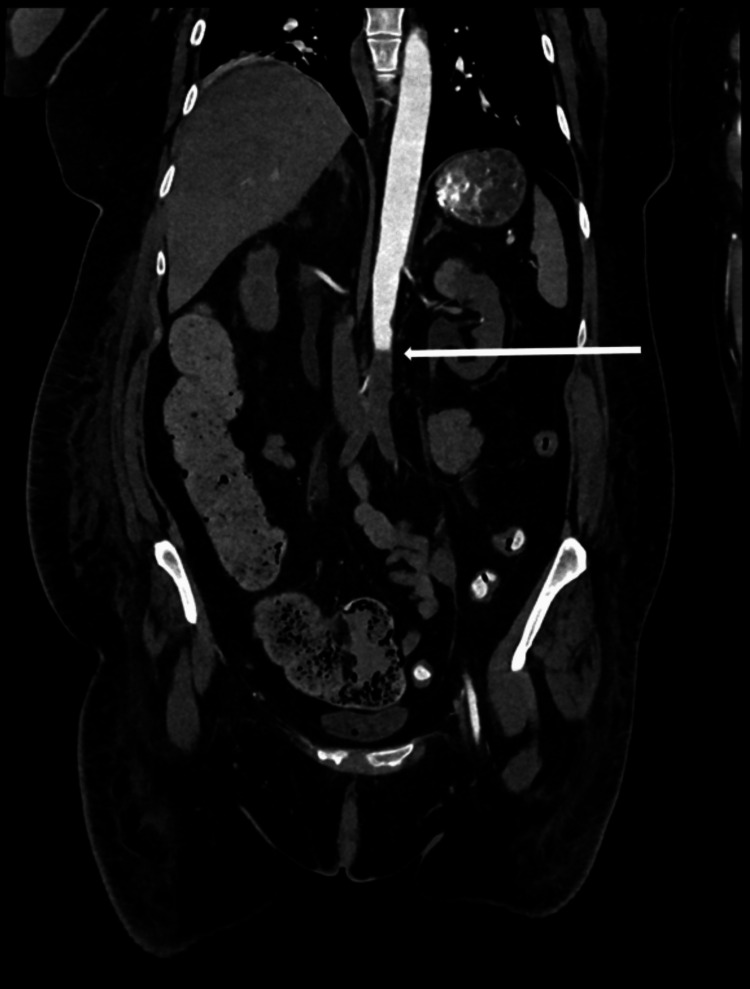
CT aorto-iliofemoral with runoff sagittal view showing an abrupt filling defect of the infrarenal aorta, consistent with acute intraluminal thrombus without distal reconstitution (white arrow).
